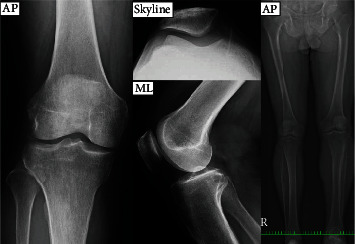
Valgus knee and patellar instability appeared on a plane X-ray with a large Q angle: 17°, tilt angle: 21°, and lateral shift: 18%.
